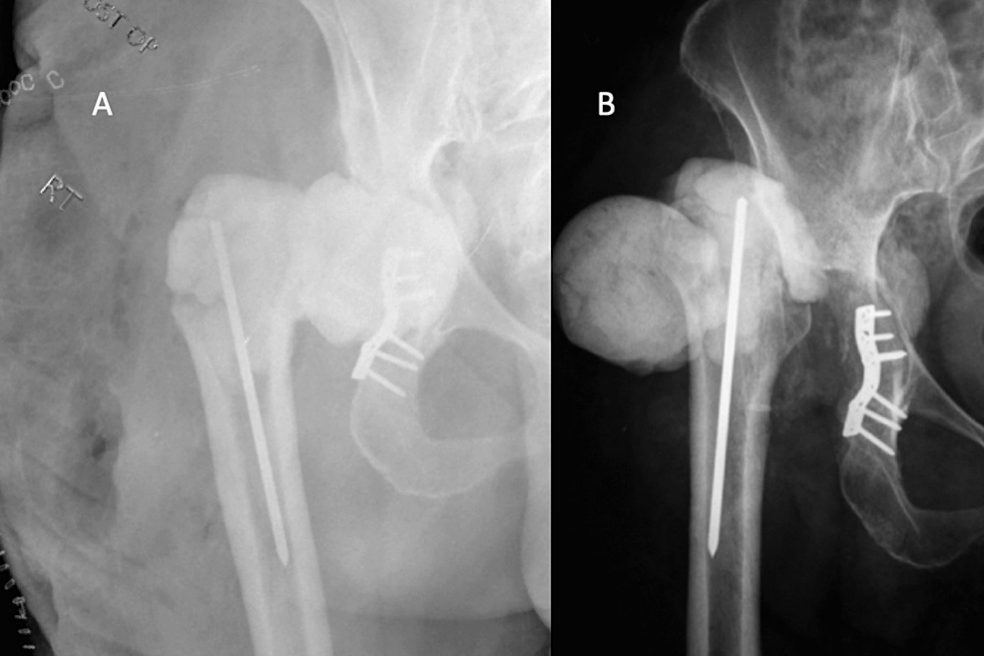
Static hip spacer mechanical complication Immediate postoperative (A) and three-week postoperative (B) anteroposterior radiographs of the hip demonstrating complete dislodgment of the acetabular component and subluxation of the femoral component
